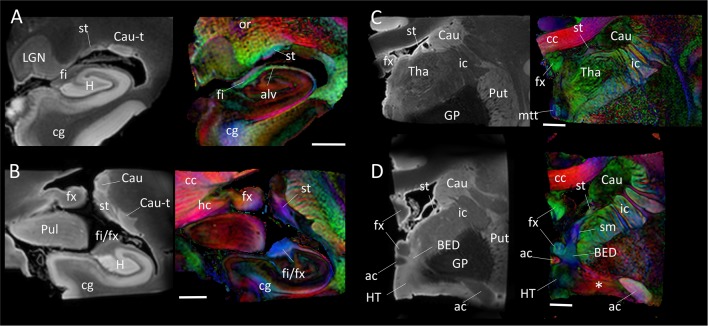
Trajectory of the stria terminalis. Panel (A–D) show high-resolution MR images at four different locations indicated in Figure 1. The stria terminalis (st) and the fornix (fx) have spatially similar, but anatomically distinct, trajectories. For the entire trajectory, the stria terminalis remains in close proximity to the caudate. Compared with the fornix, the poorly myelinated stria terminalis has lower fractional anisotropy (FA) value and higher T2 intensity, more similar to that of gray matter structures. Scale bars, 5 mm. Abbreviations are the same as in Figure 2, with the addition of: Cau, caudate; Cau-t, tail of caudate; HT, hypothalamus; LGN, lateral geniculate nucleus; Pul, pulvinar; Tha, thalamus; cc, corpus callosum; cg, cingulate; ic, internal capsule; mtt, mammillothalamic tract; or, optic radiation; sm, stria medullaris.
